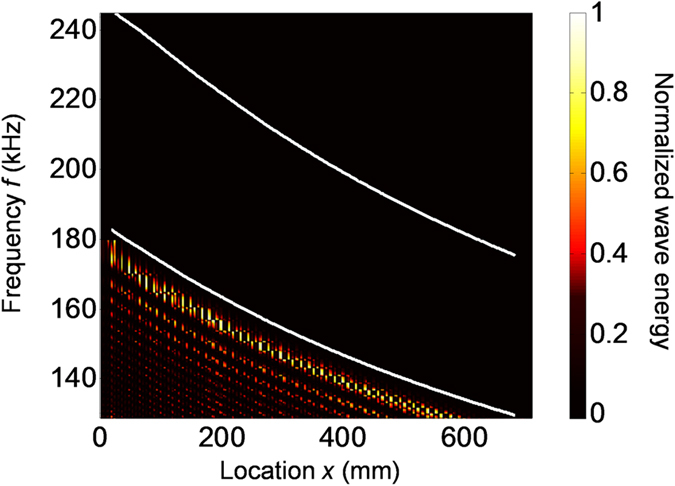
The wave energy distribution with respect to the frequency *f* and location *x*. This figure clearly shows the rainbow trapping of ultrasonic guided waves. Waves at different frequencies stop propagating forward and the corresponding wave energy is concentrated at different locations near the lower boundary of the local stopband. The two white curves represent boundaries of the local stopband obtained from Fig. 2b.
